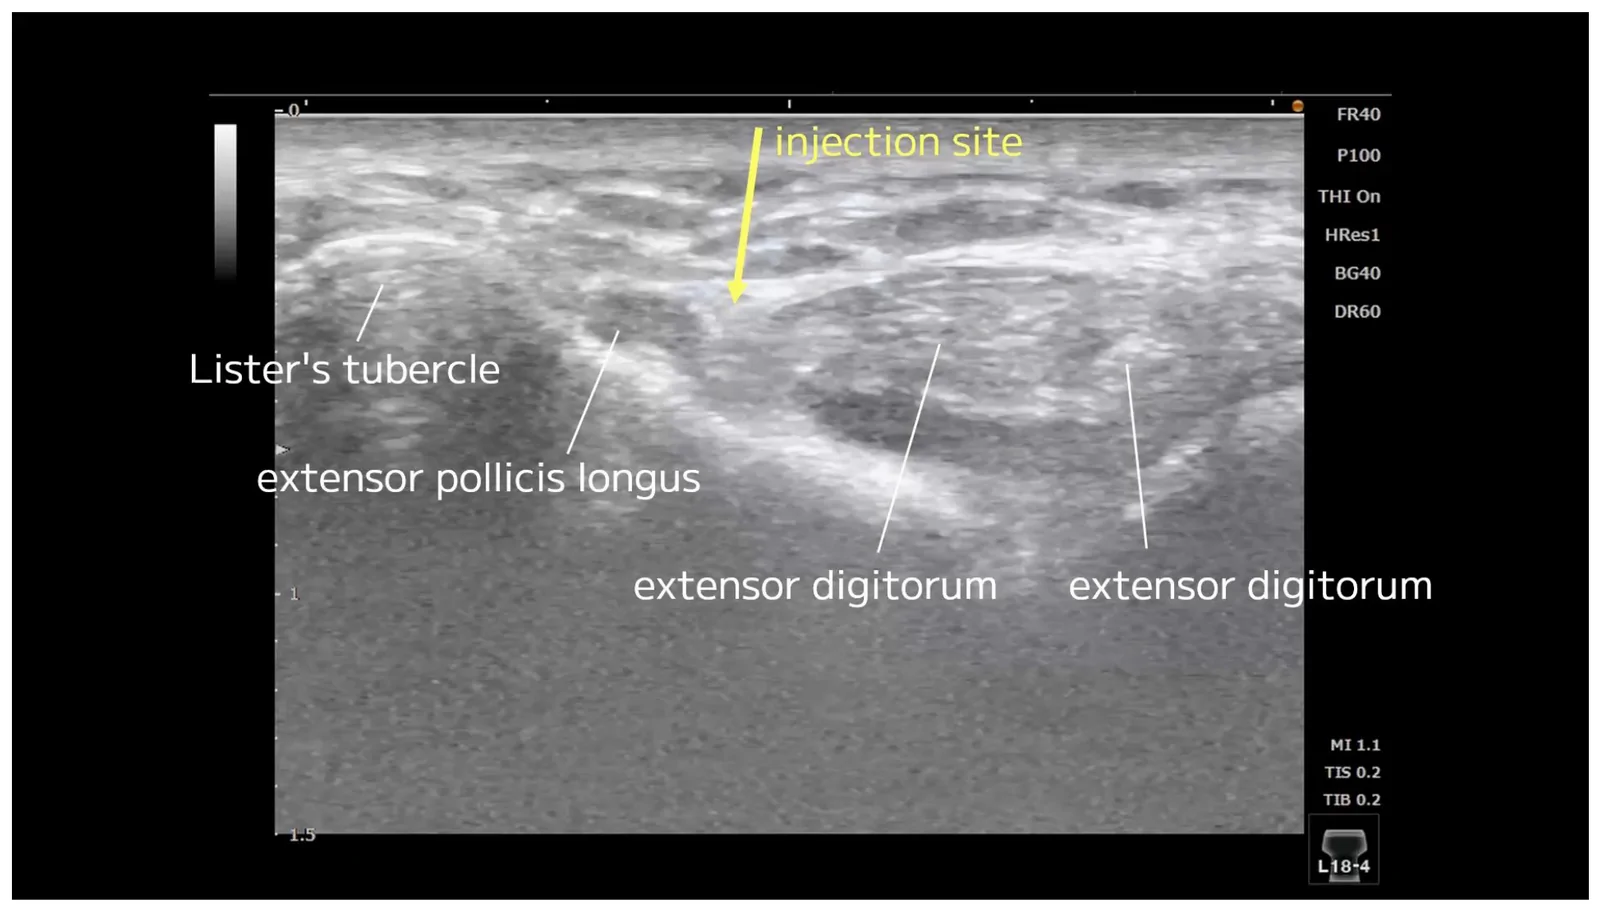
Point 2—third extensor compartment (EPL): ultrasound-guided fascia hydrorelease; yellow arrow indicates the injection site.
